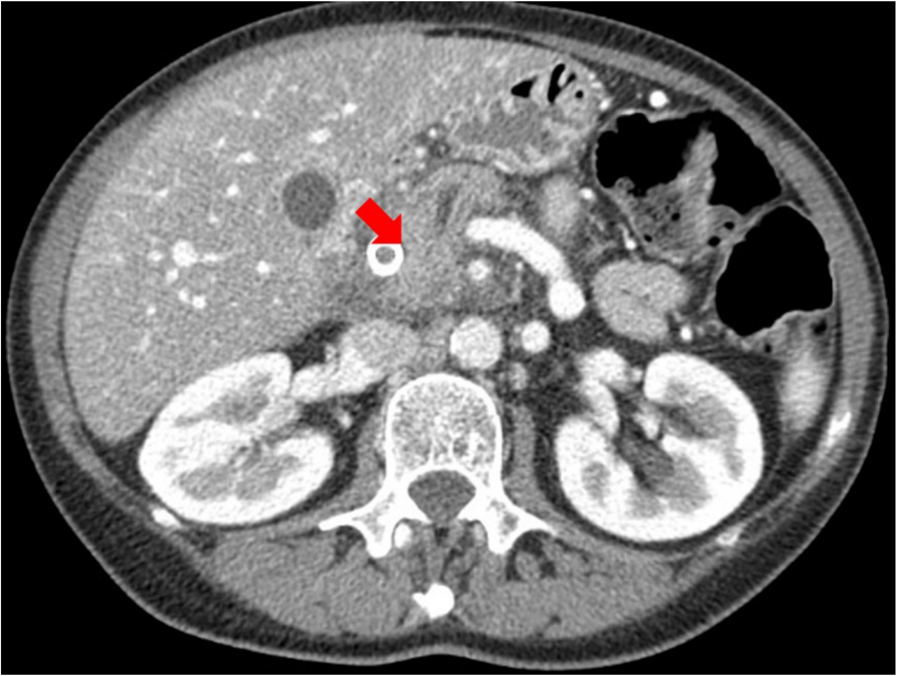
CT scan in axial plane during pancreatic phase of dynamic contrast study. Isodense pancreatic adenocarcinoma (arrow)
